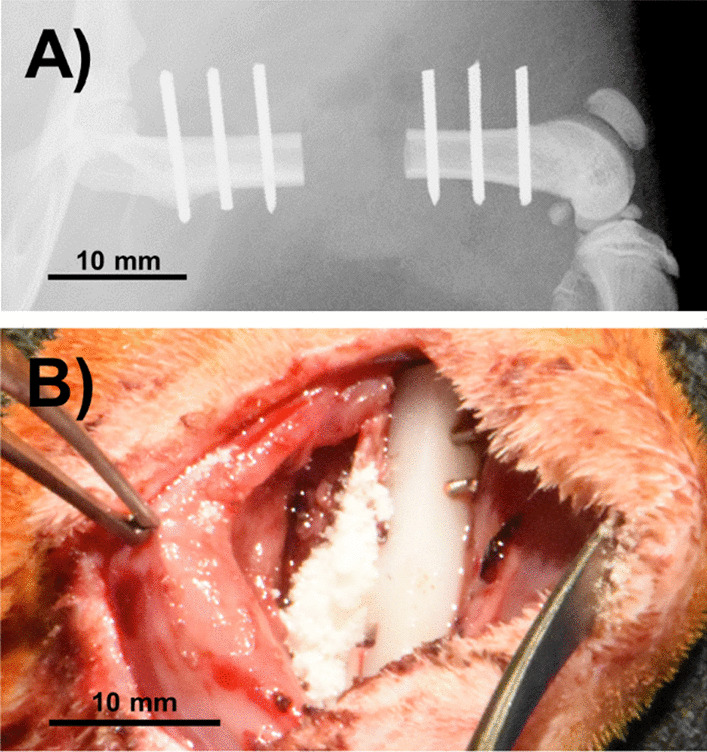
A Radiographic representation of the plate and wire placement in a 6 mm defect model. B Placement of the locally applied vancomycin powder into and immediately surrounding the 6 mm defect in the femur. Scale bar equal to 10 mm
